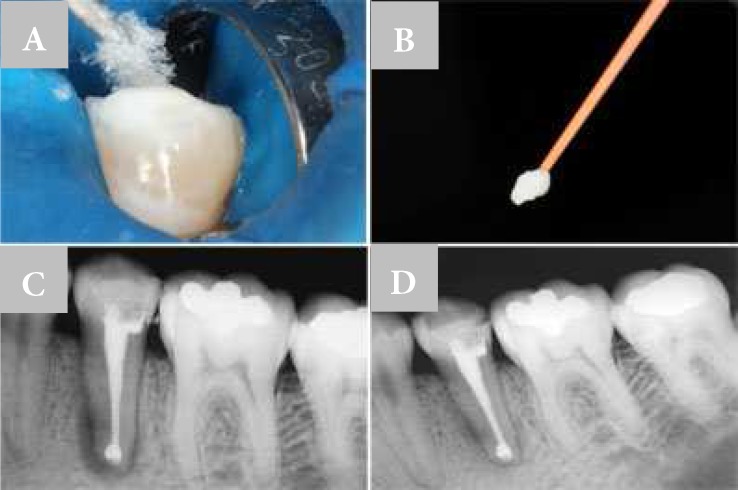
A) Insertion of lyophilized collagen sponge in the root canal; B) MTA cement insertion method; C) Immediate root canal filling with apical barrier; D) Six-month follow-up radiography
